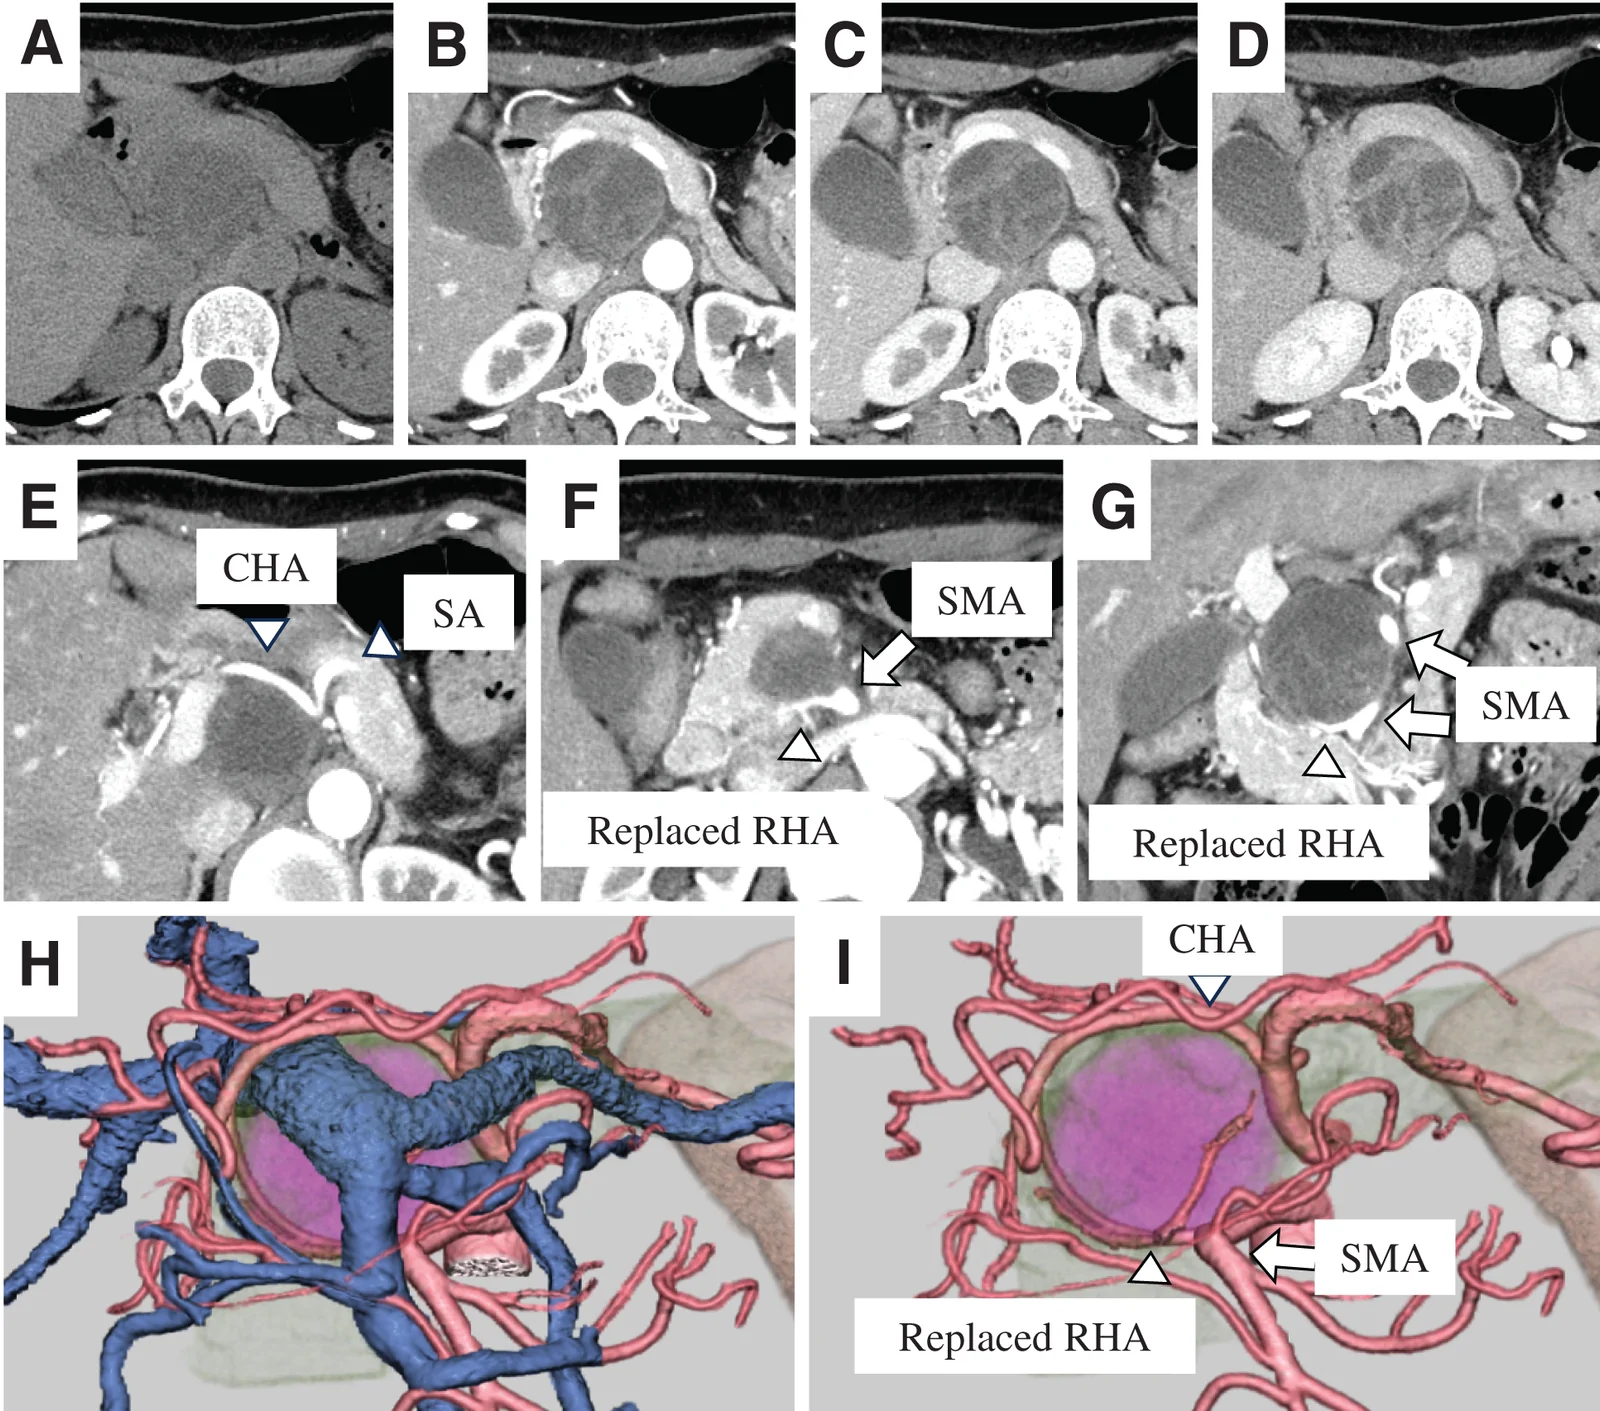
Contrast-enhanced CT and 3D vascular reconstruction findings. (A–D) Axial CT images obtained during the unenhanced (A), arterial (B), portal venous (C), and equilibrium (D) phases showing an approximately 5-cm, well-demarcated multilocular cystic mass adjacent to the pancreatic head. The lesion compresses the pancreatic parenchyma and the porto-mesenteric venous axis anteriorly and contains internal septa and focally thickened areas showing gradual enhancement across the dynamic phases. (E) Axial contrast-enhanced image showing marked displacement and compression of the CHA by the tumor. (F) Axial contrast-enhanced image showing displacement and compression of the SMA and its branch, the replaced RHA, by the tumor. (G) Coronal reconstructed image demonstrating the same findings as in (F), showing displacement and compression of the SMA and the replaced RHA by the tumor. (H, I) 3D vascular reconstruction images demonstrating the close anatomical relationship between the tumor and the peripancreatic vessels, including the CHA, SMA, replaced RHA, and porto-mesenteric venous axis. CHA, common hepatic artery; RHA, right hepatic artery; SA, splenic artery; SMA, superior mesenteric artery
